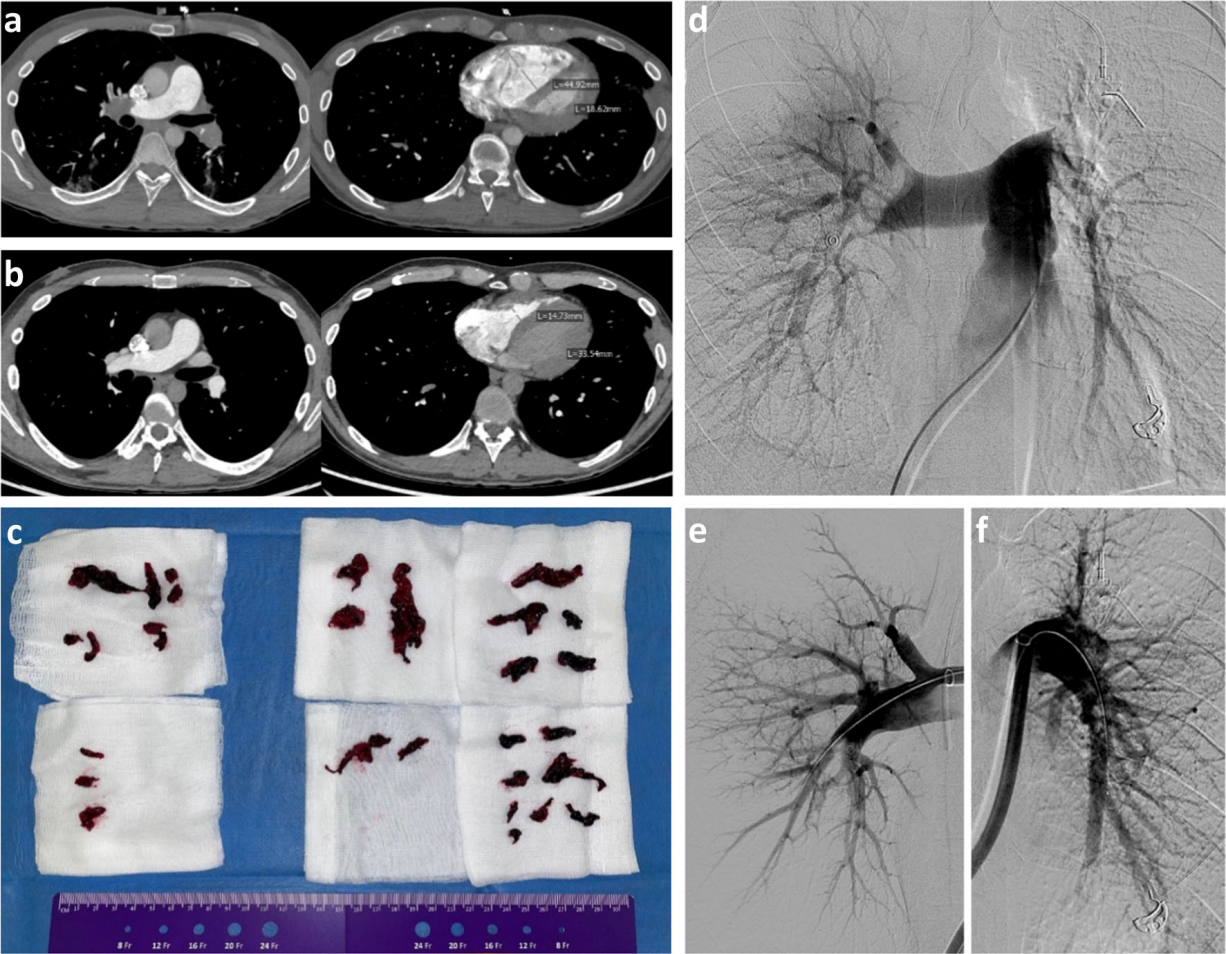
CT and angiograms from a representative thrombectomy case. Images of a 31-year-old male patient undergoing chemotherapy for colorectal carcinoma. Contrast-enhanced CT at the level of the pulmonary arteries (left) and at the level of the ventricles (right) before (a) and 1 week after thrombectomy (b) with the 24F device. The right ventricle is decompressed after successful thrombectomy. Right-to-left ventricular ratio improved from 2.4 to 0.43. Digital subtraction angiograms before (d) and after (e, f) thrombectomy. Extracted clots are shown in (c). The mean pulmonary artery pressure dropped from 35 to 16 mmHg, and the heart rate decreased from 130 to 85 beats per minute. Intensive care unit duration of stay was 2 days, hospital stay was 7 days
